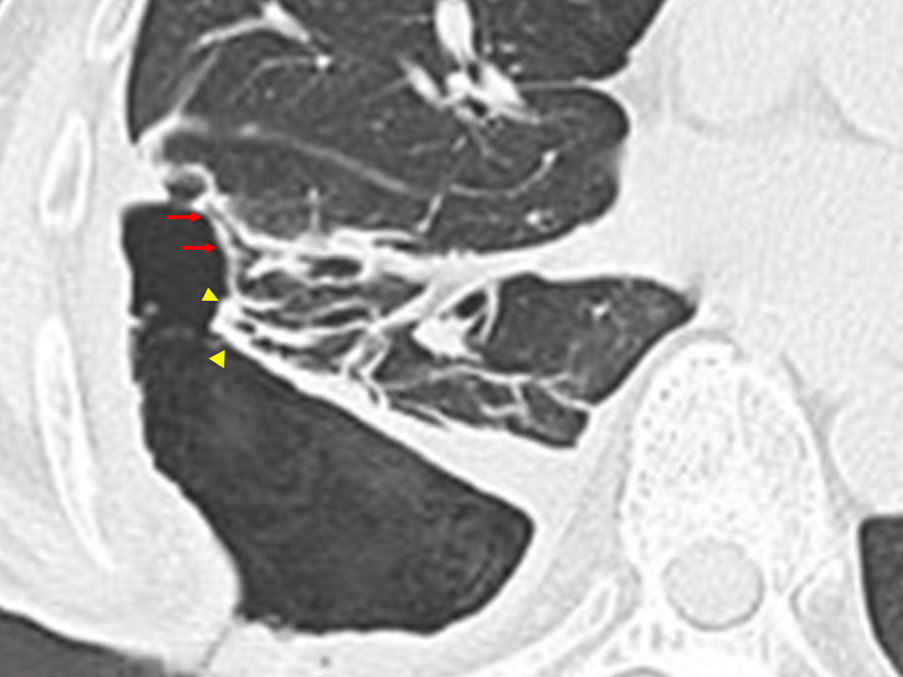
Computed tomography of the chest at 1.5 months after the thoracostomy. The subpleural lung parenchyma in the right B8 (arrows) and B9 (arrowheads) regions is completely desquamated and numerous bronchial fistulas have appeared.
